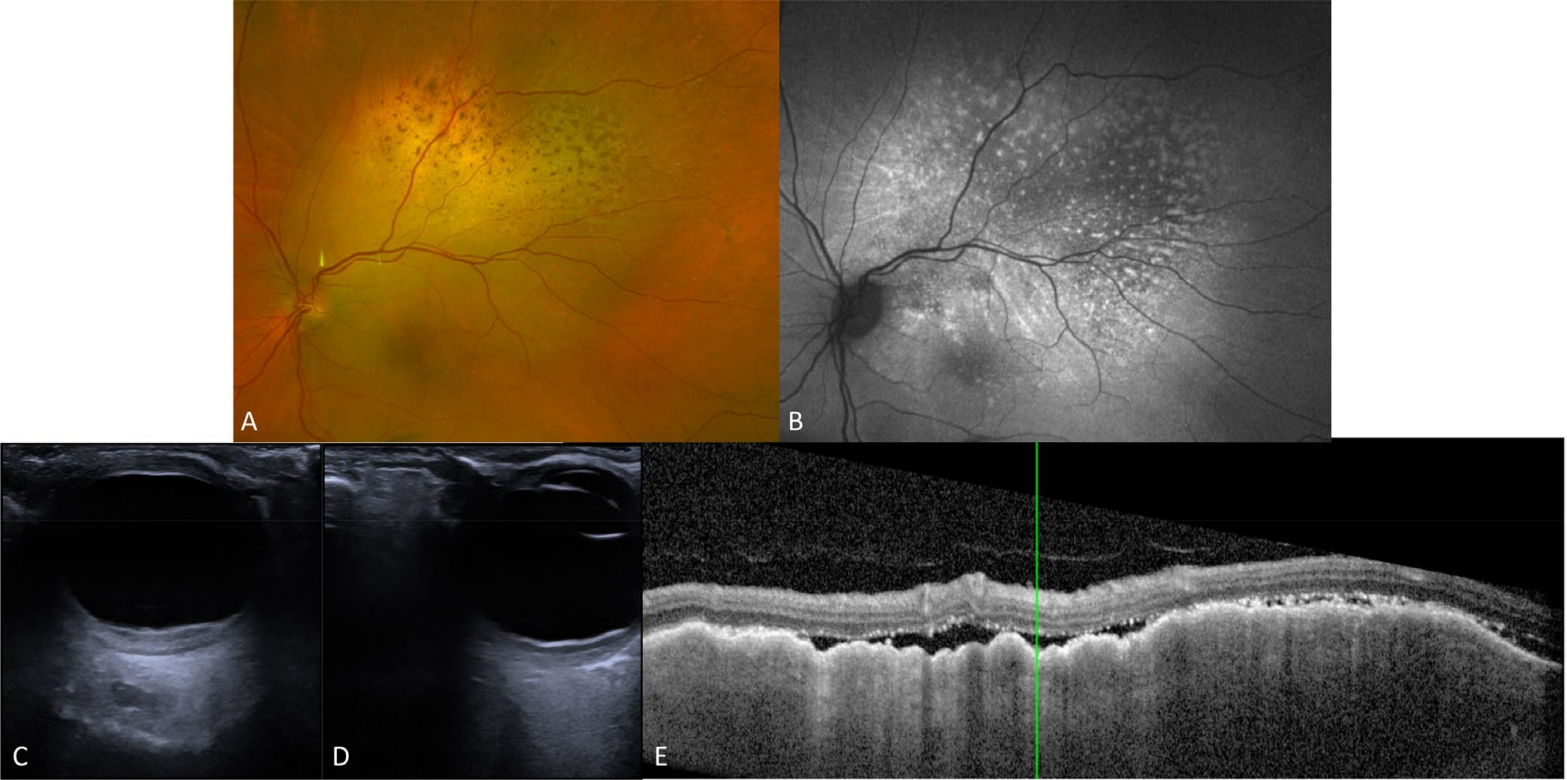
Choroidal metastasis secondary to lung cancer. On pseudocolour (A) and autofluorescence (B) images, there is a yellowish choroidal lesion with overlying lipofuscin. On high resolution ultrasound (C and D), it has medium internal echogenicity, with transverse base 12.8 mm, longitudinal base 14.6 mm and elevation 1.1 mm. The OCT scan (E) shows choroidal thickening with lumpy bumpy appearance and overlying subretinal fluid and trace of lipofuscin. Patient consent obtained for the use of images for publication.
